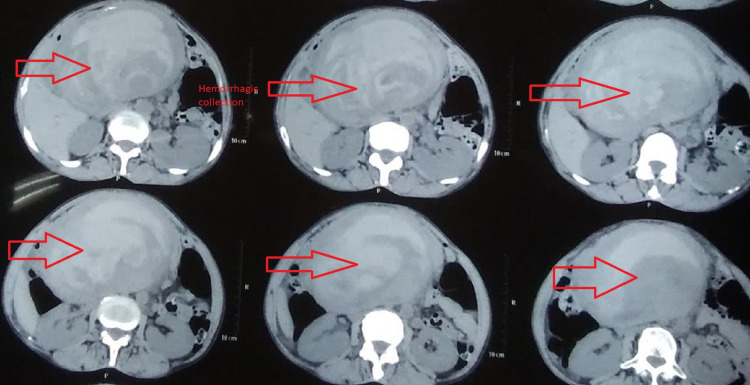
CT: Transverse sections showing retroperitoneal blood collection. CT: computed tomography
